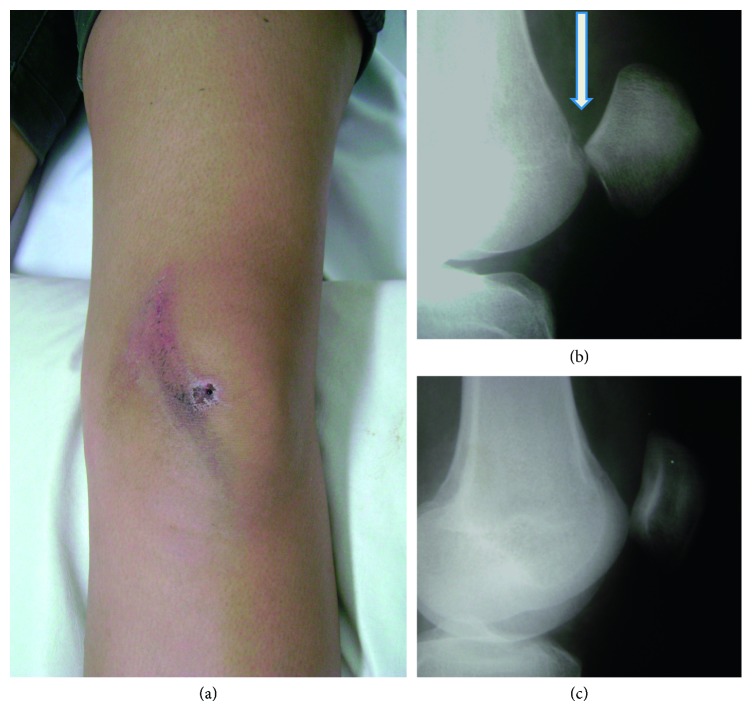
Image shows the patient's left knee upon arrival at the ED, after a fall from a bicycle (a). It is possible to notice that there is no bulging in the anterior region of the knee found in the VDP. SDP is suspected based on the attachment of the distal end of the patella to the proximal end of the trochlea (arrow). The high riding patella needs to be distinguished from a patellar tendon rupture (b). Radiograph of the left knee obtained after the procedure shows successful reduction (c). ED: emergency department; SDP: superior dislocation of the patella.
